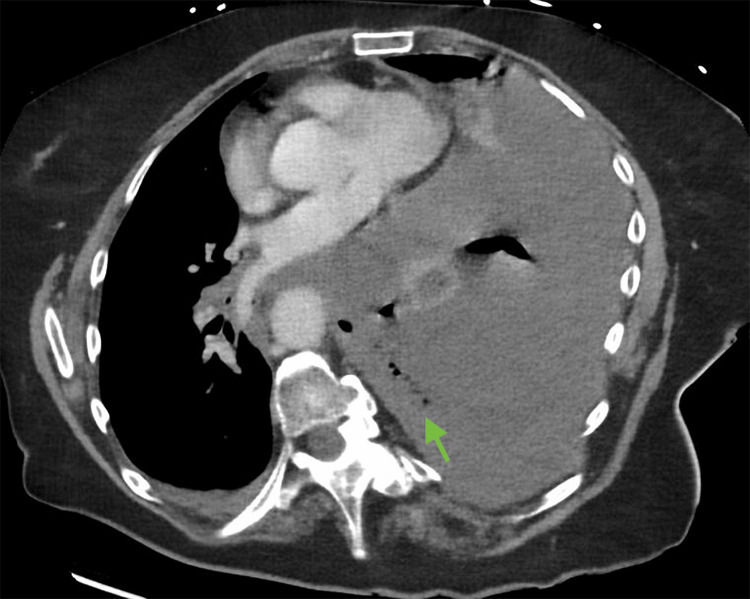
Axial contrast-enhanced CT image Axial contrast-enhanced CT image demonstrating the herniated stomach within the thoracic cavity. Air bubbles are visible within high-attenuation pleural fluid in the left hemithorax (green arrow), suggestive of perforation with associated hemorrhagic effusion.
